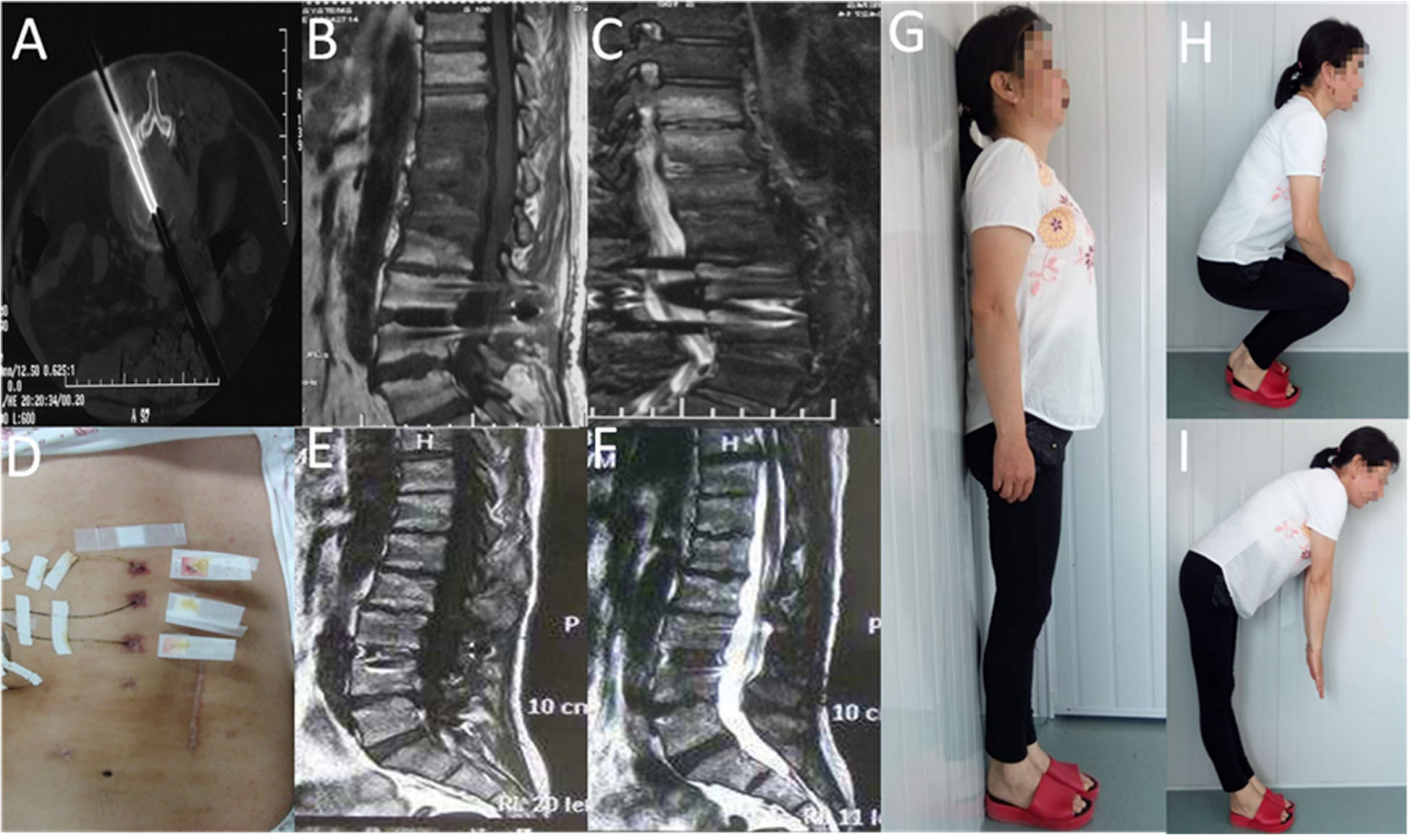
The same 40-year-old female as shown in Fig. 2. Samples confirmed a fungal spondylodiscitis (case 1) using intraoperative CT-Guide(a). At five months after the minimally invasive debridement and irrigation, MRI showed that the L2-5 infections had resolved but the infection had progressed to the L1-2、T12-L1 and T11-12 levels (b and c). Postoperative minimally invasive debridement and irrigation picture(d). At the 48-month follow-up, MRI (e) and (f) showed all the infections had resolved and no further progression was observed; T1-weighted image showed increased signal with the extensive additional enhancement of the fat signals of vertebral bodies. The endplates were grossly deformed. T1 and T2-weighted show a narrow line at the site of the discs
